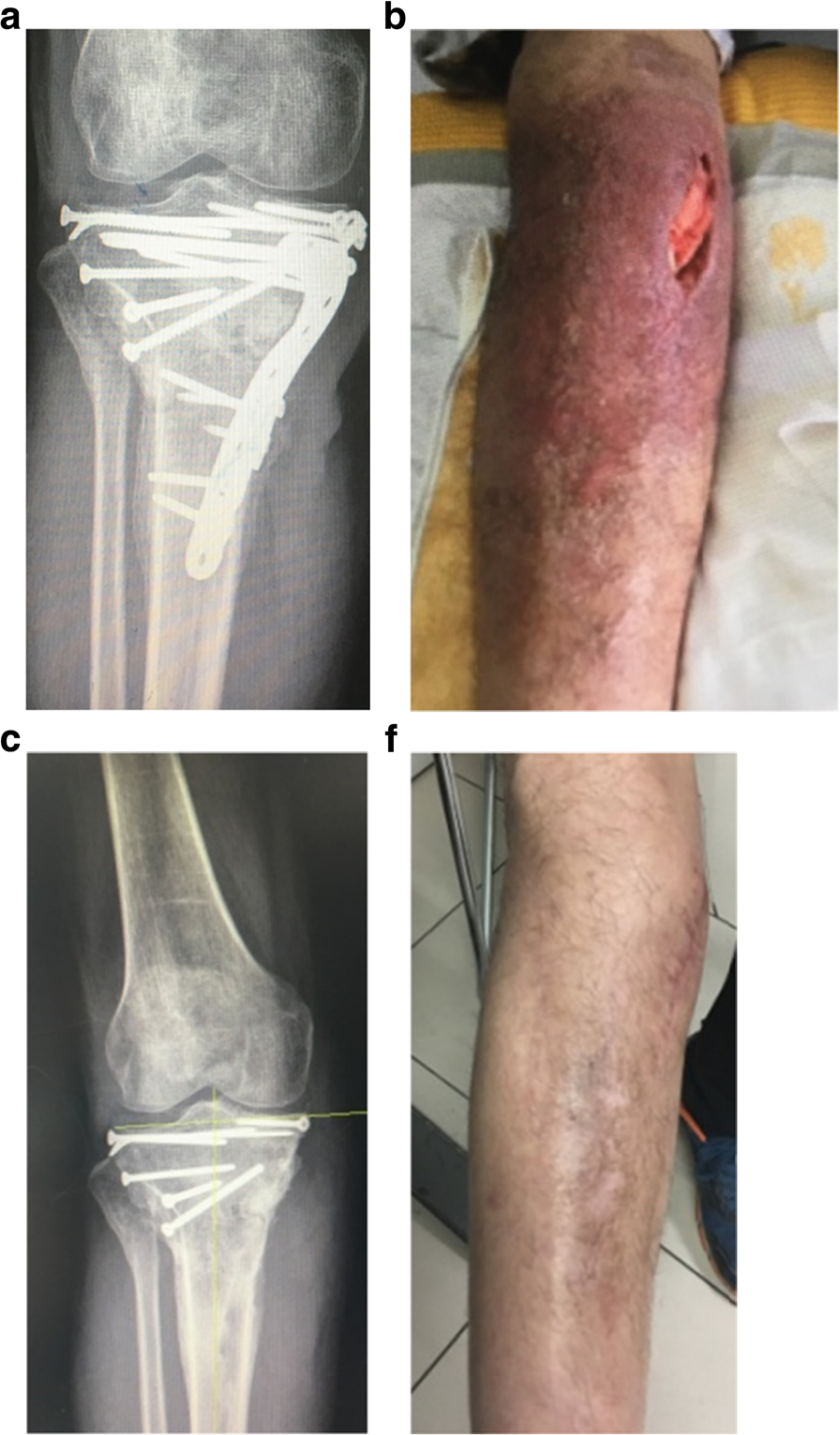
Stable hardware + bone healed = remove hardware, debridement, soft-tissue coverage. a Radiographs of primary internal fixation. b Partial exposure of the wound. c The plate was removed. d After 24 months of treatment, the wound was stable
